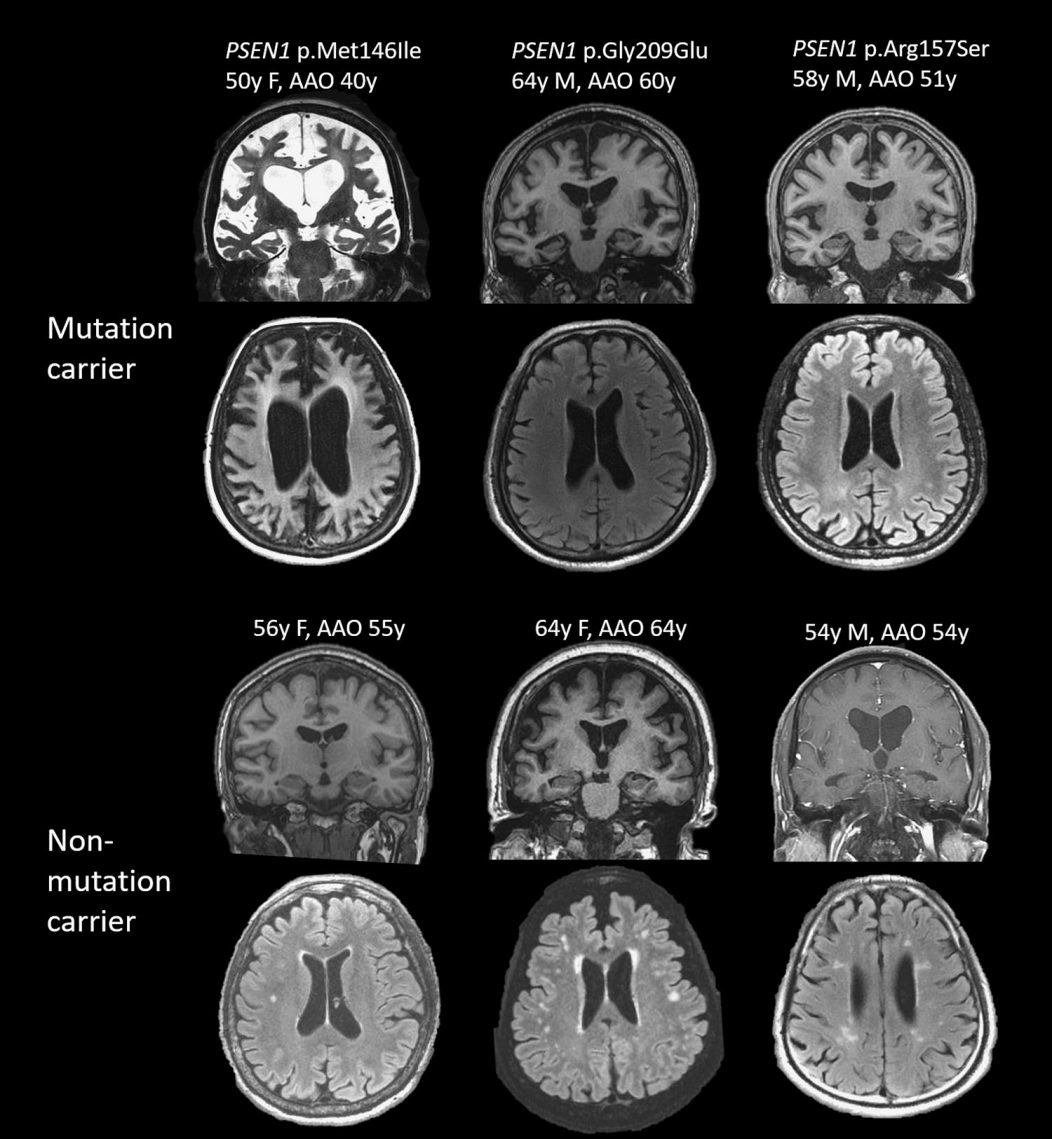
Representative MRI of each of 3 age-matched mutation carriers and non-carriers. Coded in the order of scale of medial temporal atrophy, posterior atrophy (PA) and white matter hyperintensity (WMH), from left to right in mutation carriers are (4,3,0), (2,1,0) and (1,2,0) and non-carriers are (1,1,1), (1,1,2) and (3,0,2). Various degree of MTA was noted across patients with or without mutation; in the contrary, WMH was seen predominantly in non-mutation carriers.
